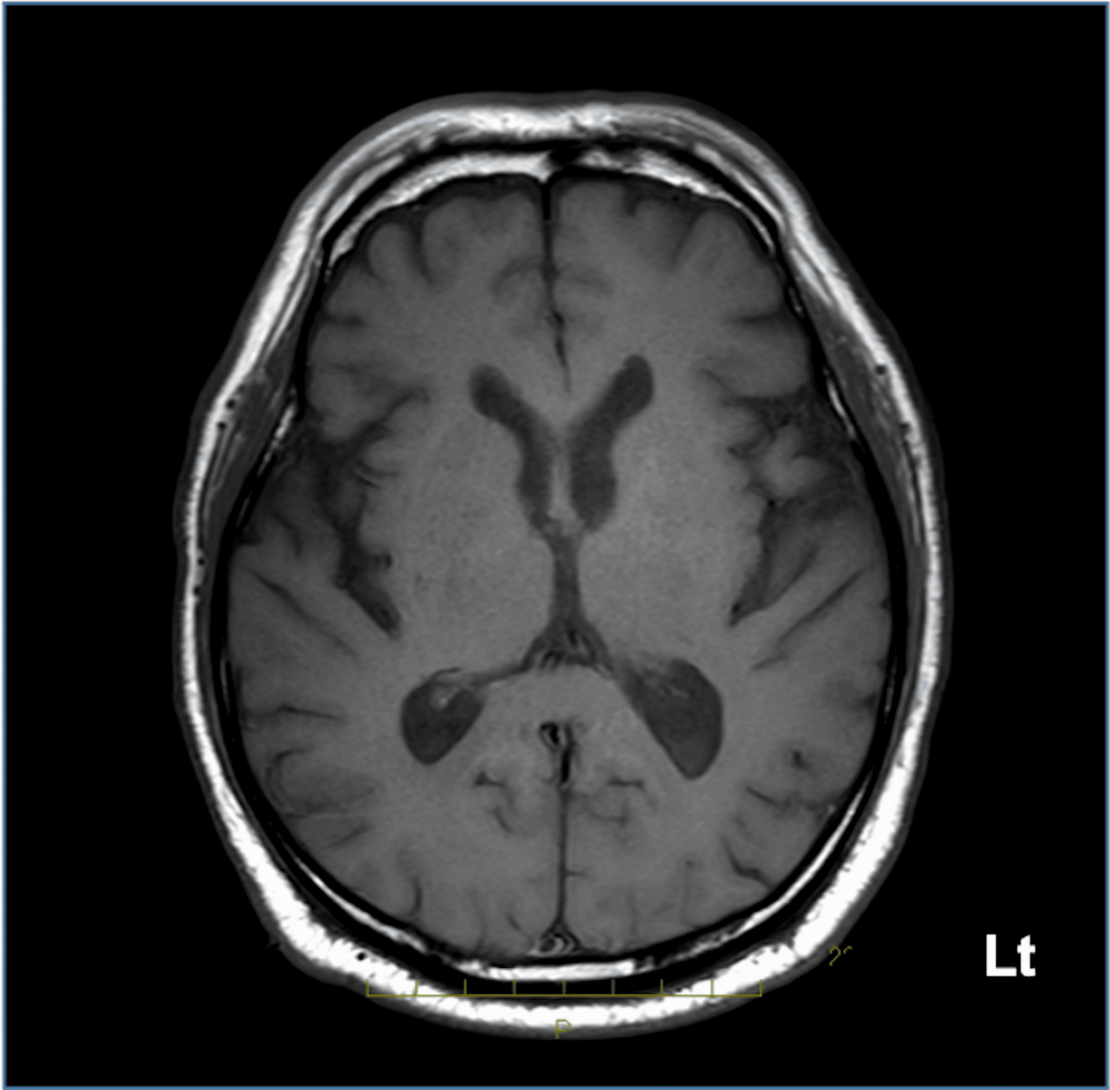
T1-weighted brain MRI of the same cross-section from one year ago A ventricular enlargement is not observed.
